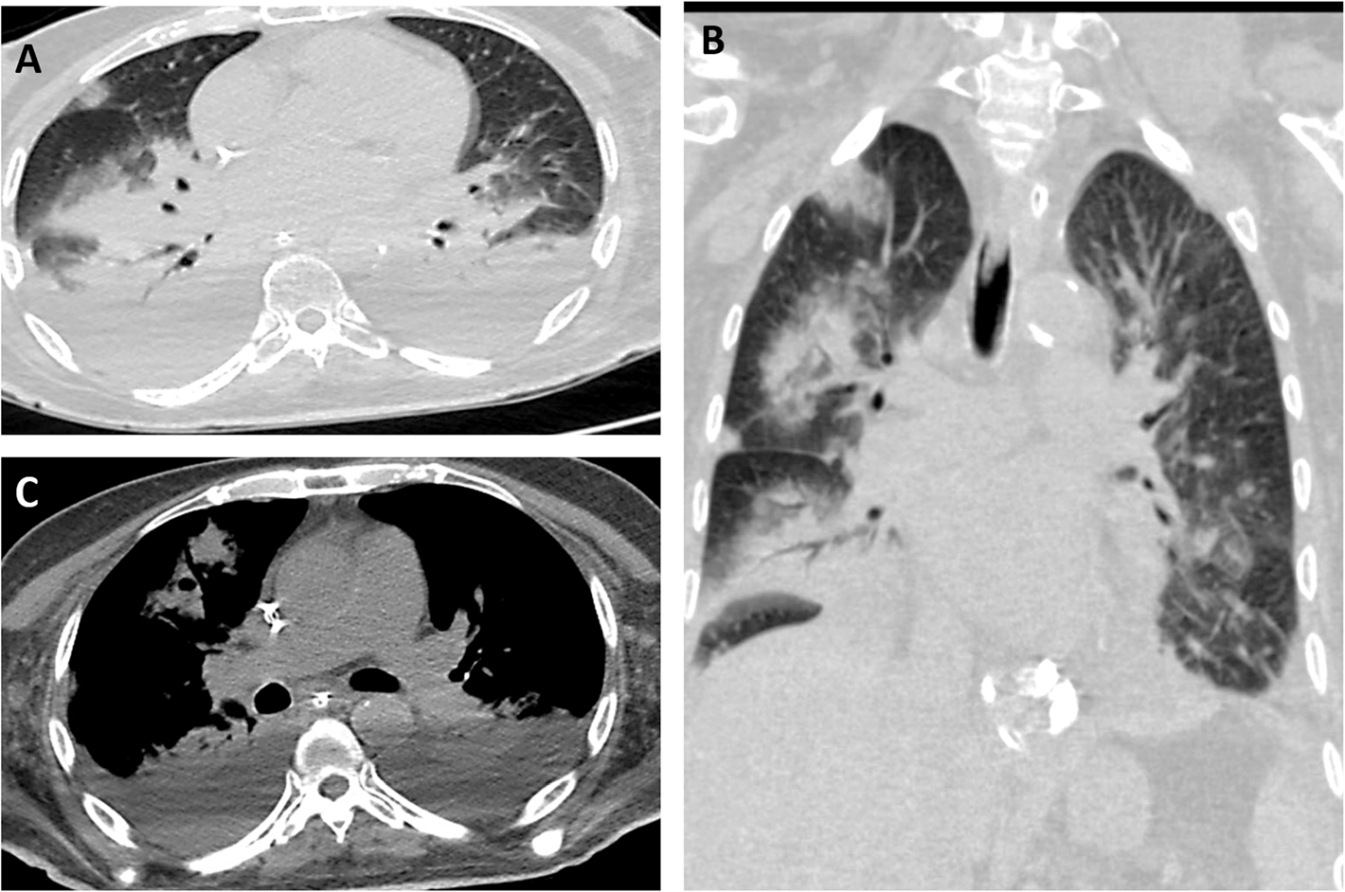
Non-contrast CT done 5 days after initial complaint of a 60-year-old male with fever, cough, body aches, and shortness of breath. rt RT-PCR +ve (A-C): A, axial and B, coronal lung window and C, axial mediastinal window show bilateral perivascular consolidation (organizing pneumonia pattern) with air bronchogram sign involving both middle and lower lobes with bilateral pleural effusion
